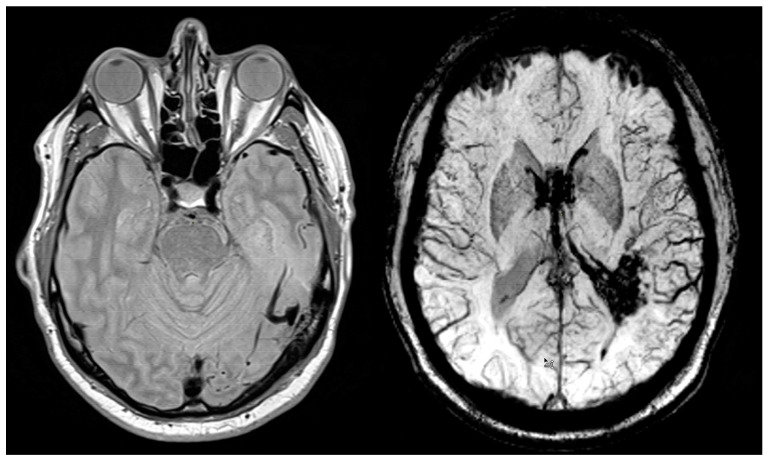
Magnetic Resonance Images, Axial View: The images reveal a cerebral arteriovenous malformation with tortuous vessels. Proton density sequence T2-weighted susceptibility-weighted imaging sequence.
